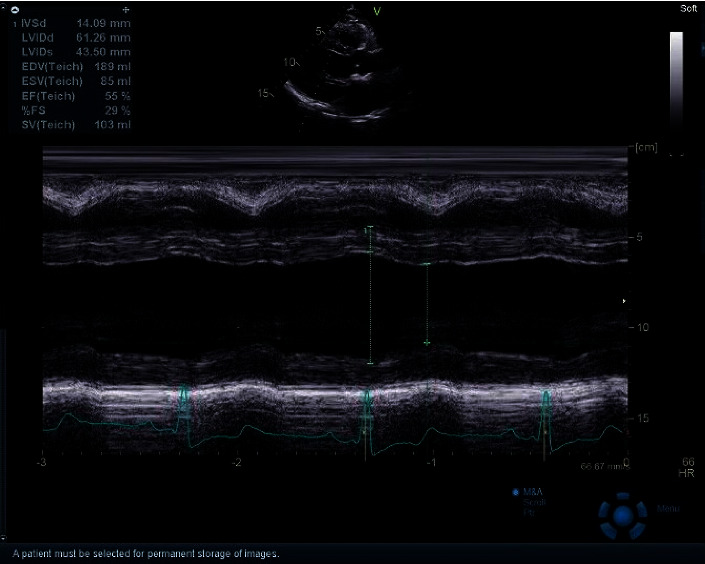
Echocardiographic analysis of left ventricular mass.
